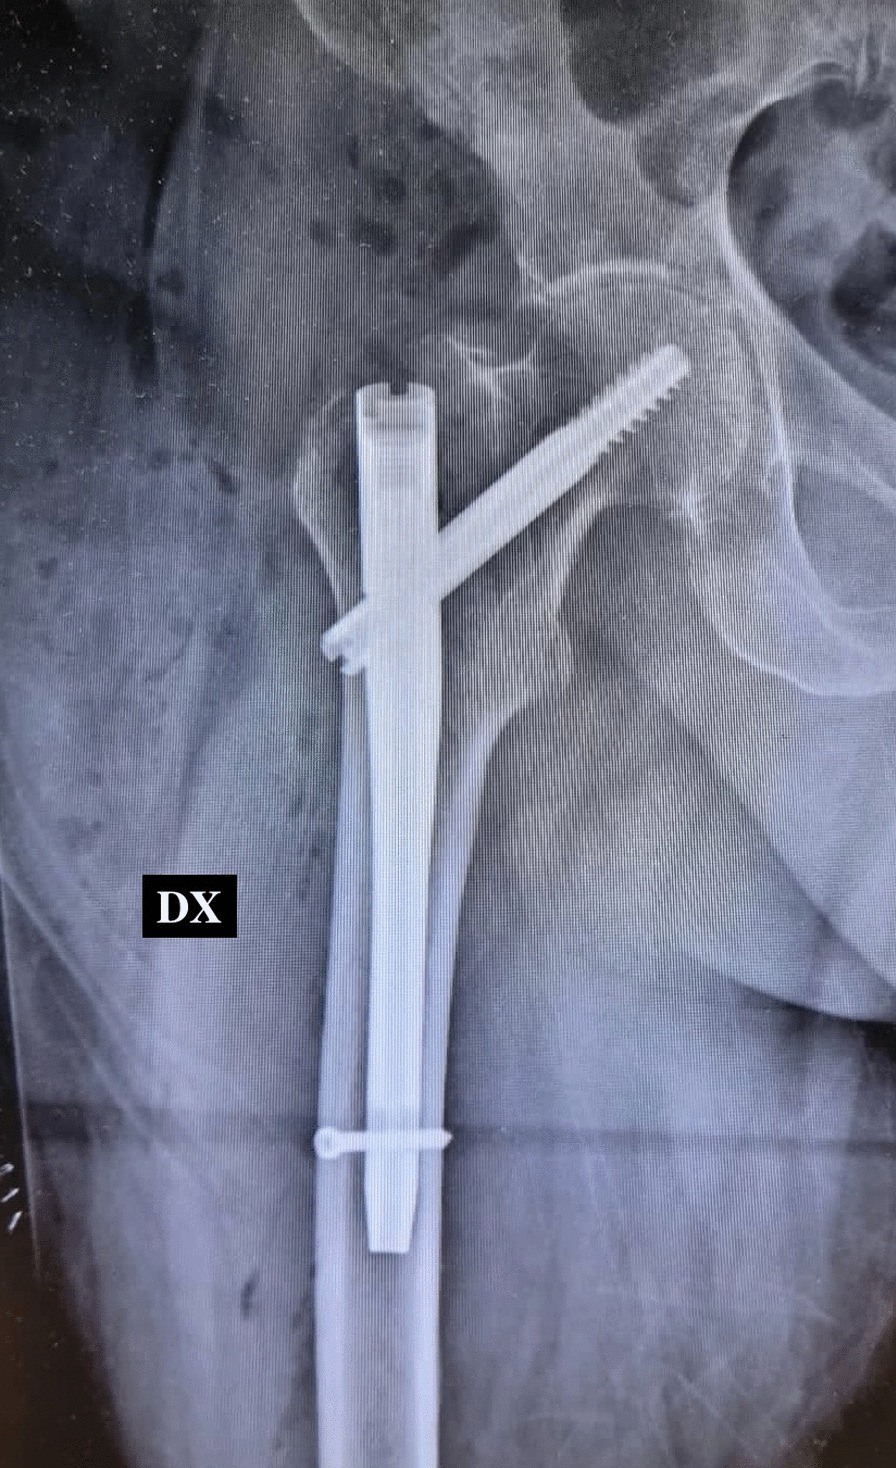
Postoperative anteroposterior radiograph of the right hip
